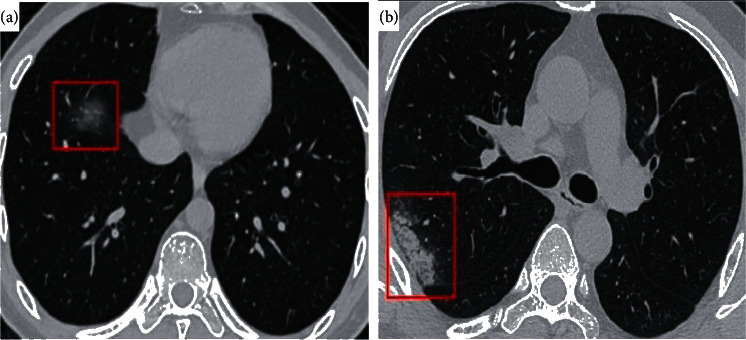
(a) Chest CT scan image shows a pure ground-glass opacity (GGO) in the right lower lobe (red frame). (b) Consolidation in the right lobe subpleural area (red frame).
